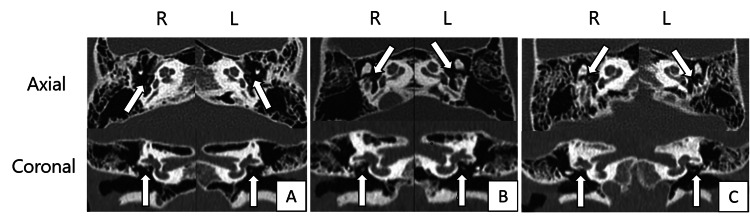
Temporal bone computed tomography images for Case 1 (A), Case 2 (B), and Case 3 (C). Axial images are shown in the upper panels, and coronal images are shown in the lower panels. (A) Case 1 shows bilateral absence or hypoplasia of the long process of the incus, suggesting bilateral incudostapedial discontinuity. (B) Case 2 shows bilateral defects of the long process of the incus, consistent with bilateral incudostapedial discontinuity. (C) Case 3 shows a right-sided defect or hypoplasia of the long process of the incus, whereas the left ossicular chain is morphologically normal. White arrows indicate the affected long process of the incus.
